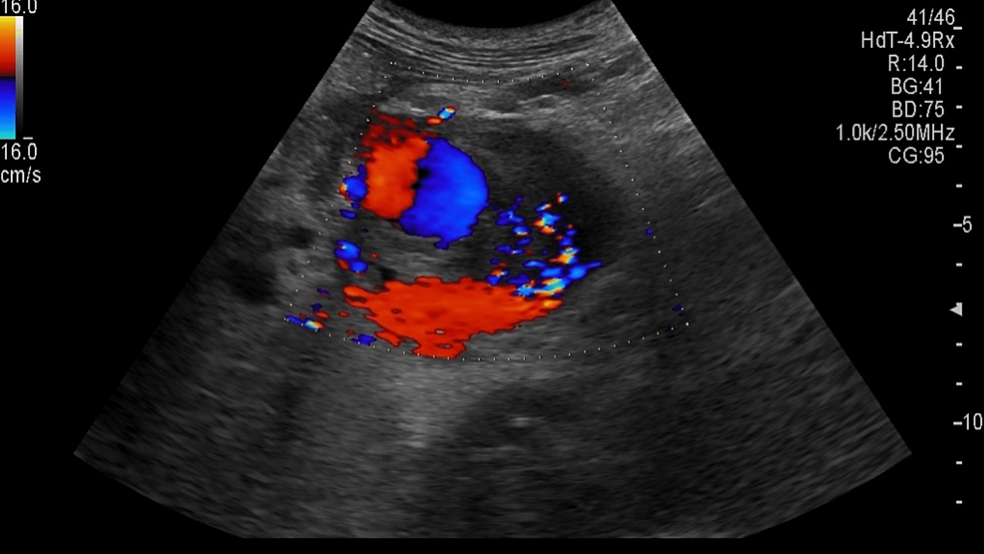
Colour Doppler of the lesion depicting the yin-yang sign of pseudo-aneurysm.
